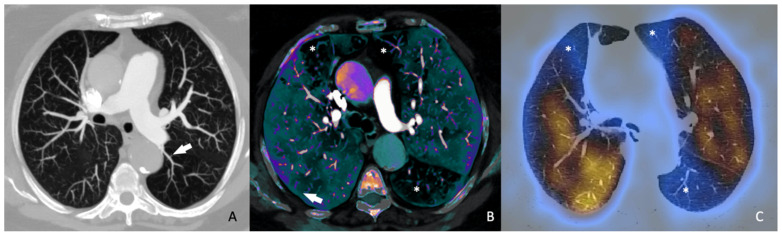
Eighty-nine-year-old woman diagnosed with chronic pulmonary thromboembolism. (A) Axial CT angiography (maximum intensity projection—MIP—reconstruction) showing severe narrowing in the superior segmental artery of the left lower lobe (white arrow) as sequela of PE. (B) Fusion image of CT angiography and color-coded iodine density showing wedge-shaped perfusion defects (*) in the middle lobe, lingula, and left lower lobe, with the latter corresponding to the findings in image (A). (C) SPECT-CT fusion image showing wedge-shaped perfusion defects (*) similar to those obtained with dual-energy CT (B).
